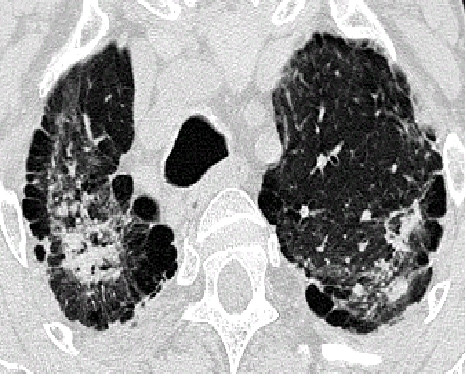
HRCT-scan of severe aluminosis with subpleural bullae.
